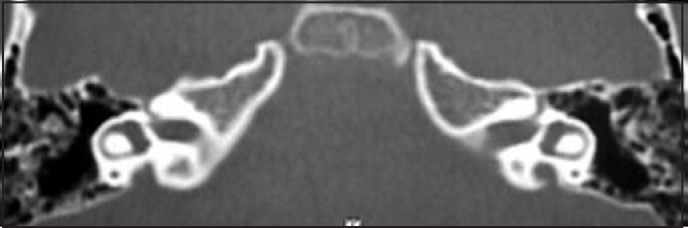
CT scan showing bilateral large vestibular aqueduct.
